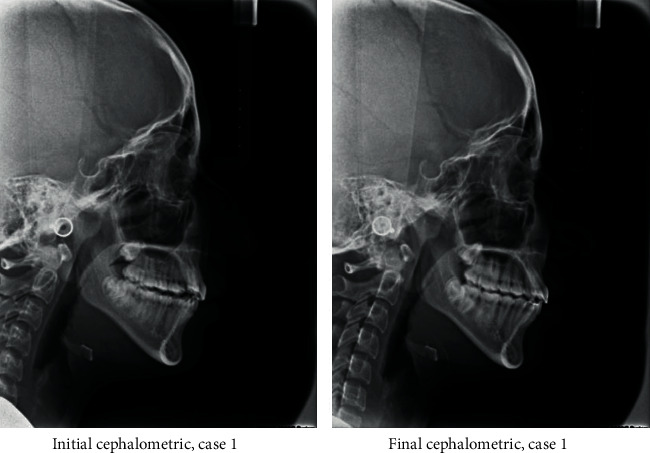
Initial and final cephalometric radiographs.
